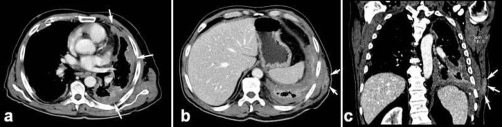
Contrast enhanced CT of the chest showing the pathological circumferential pleural thickening (a) and the chest wall tract metastasis (b, c).
